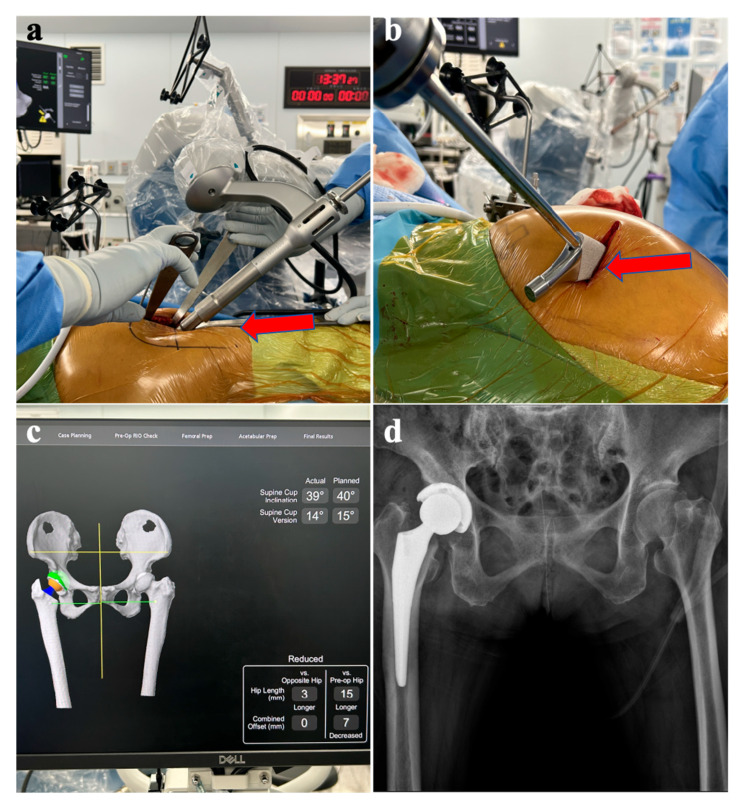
Intraoperative and postoperative images of dual-incision MAKO-assisted total hip arthroplasty. (a) Placement of the acetabular cup through the anterolateral incision using the MAKO robotic arm. The arrow indicates the surgical field where the acetabular component is being inserted. (b) Insertion of the femoral stem through the posterolateral incision. The arrow identifies the site of stem insertion. (c) Three-dimensional reconstruction displayed by the MAKO system after implantation of components, demonstrating the final implant position. (d) Postoperative anteroposterior pelvic radiograph obtained on postoperative day 2, showing satisfactory placement of the acetabular and femoral components.
